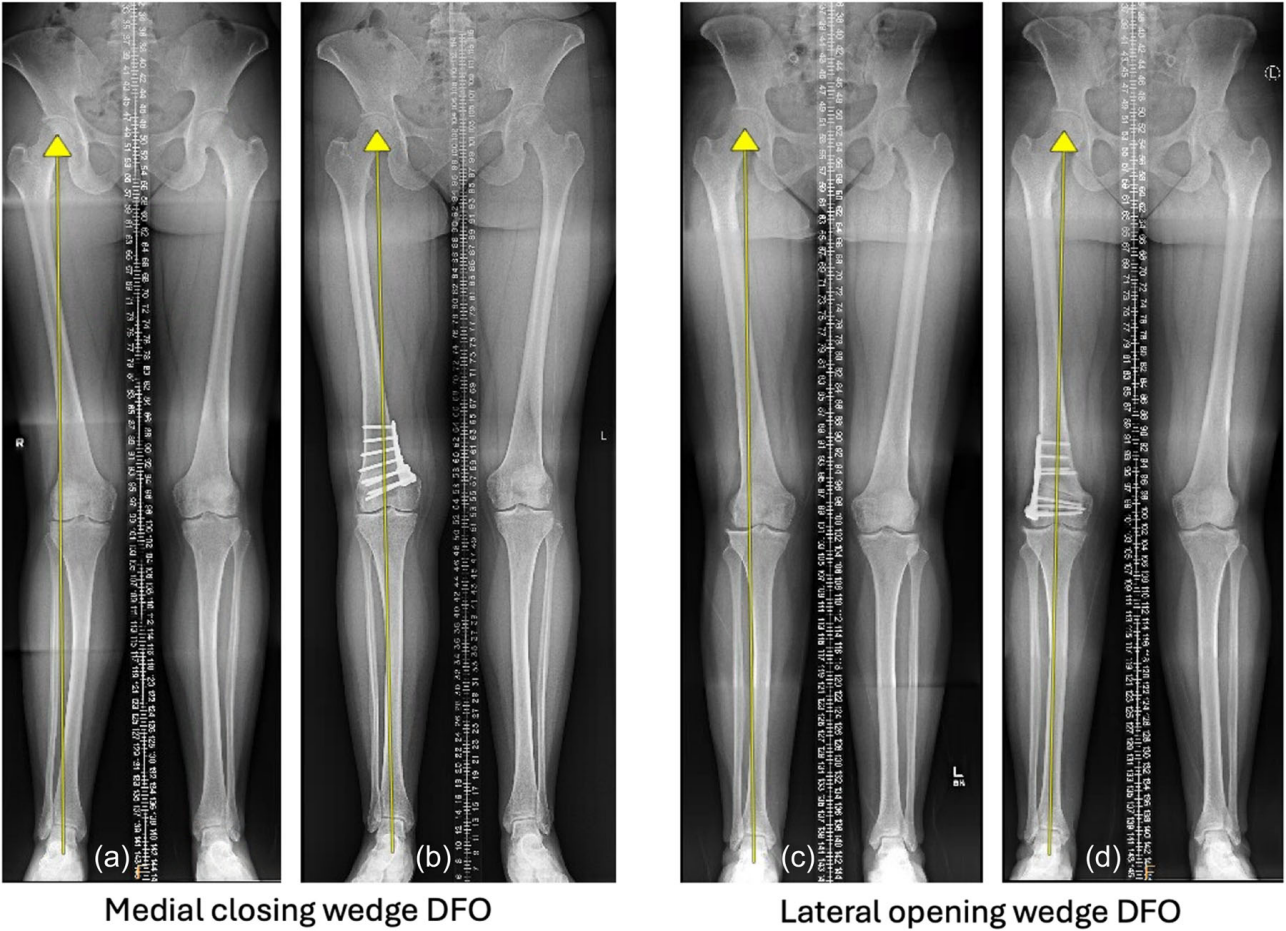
Radiographic examples of pre‐ and post‐operative distal femoral osteotomy (DFO) for correction of valgus alignment with lateral compartment disease. Panels (a) and (b) demonstrate pre‐ and post‐operative medial closing wedge DFO, respectively. Panels (c) and (d) demonstrate pre‐ and post‐operative lateral opening wedge DFO, respectively. Arrows drawn in each radiograph depict the mechanical weightbearing (Mikulicz) line.
